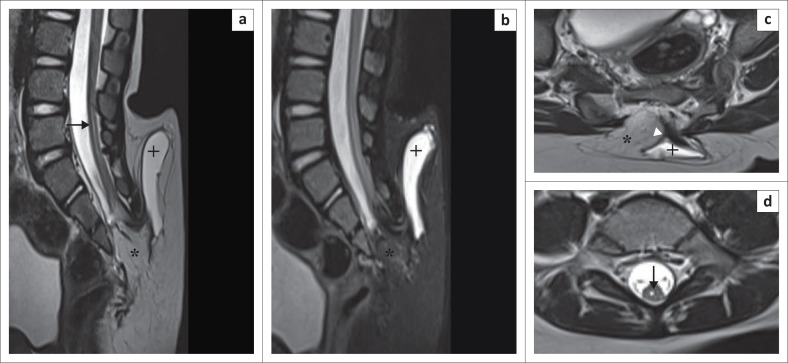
Lipomyelomeningocoele. Sagittal T2-weighted image (T2WI) (a) and sagittal short tau inversion recovery (STIR) (b) demonstrate a low-lying spinal cord tethered to a lipoma (*). Axial T2WI (c) shows protrusion of neural tissue (white arrowhead) and an enlarged cerebrospinal fluid (CSF) space (+) through the bony defect in the posterior elements. The cord–lipoma interface is present outside the spinal canal. The overlying skin is intact. Syrinx formation (black solid arrow) is noted (a, d) at the L5 and S1 vertebral levels.
